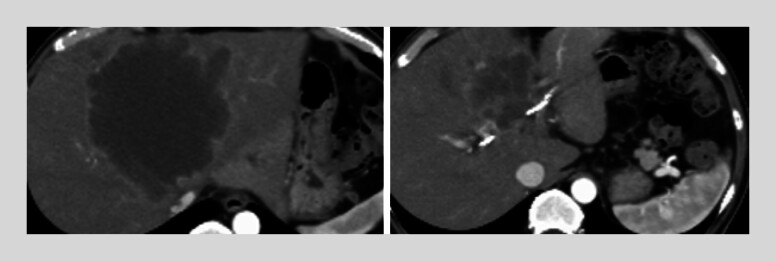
Computed tomography (CT) at presentation showing a tumor centered in hepatic segment 4 in a 59-year-old man who presented with obstructive jaundice.
